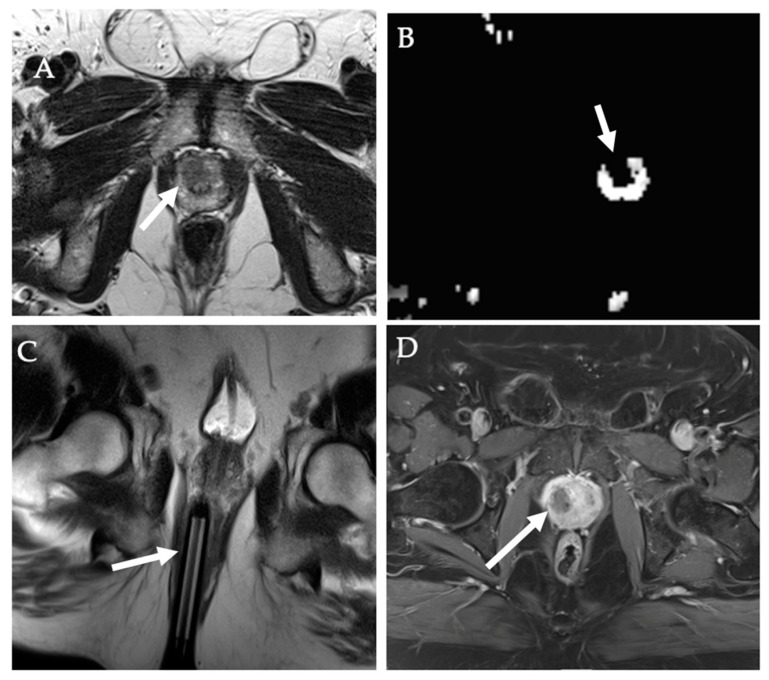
Laser ablation of prostate tumor. (A,B) Axial T2WI (A) and ADC (B) MR images show a right transition zone prostate lesion (white arrow) in a 66-year-old male with rising PSA and MR Bx positive for a Gleason score of 3 + 3 = 6 cancer. MR-compatible 18G biopsy gun deployed into the target during in-bore MR biopsy (C). (D) Subsequent MR-guided focal laser ablation was performed, with post-ablation contrast-enhanced T1WI showing complete necrosis of the target tumor.
